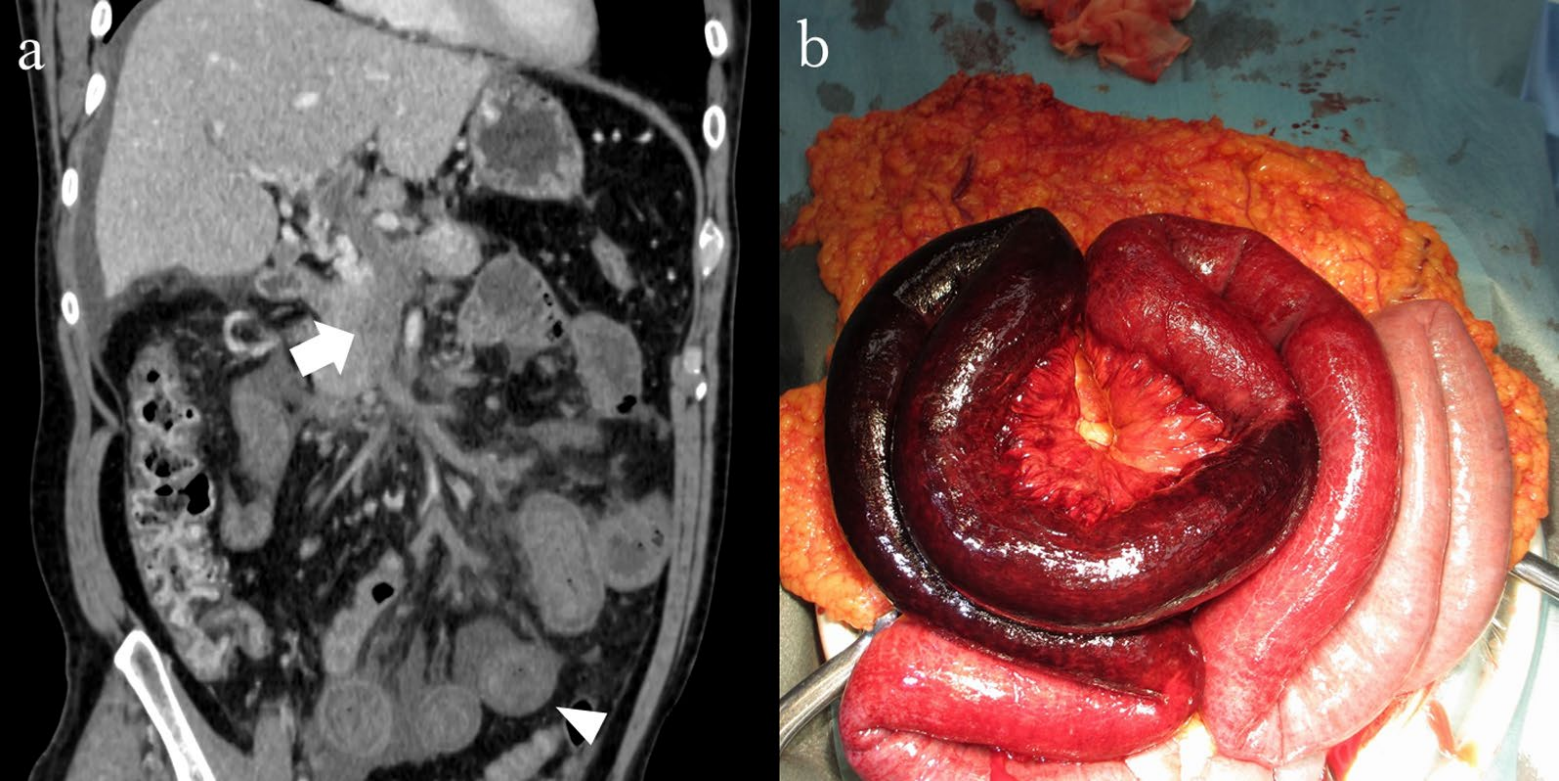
a CE-CT findings (coronal view) of Case 2. Thrombus completely occludes SMV and portal vein (arrow). Jejunal wall with poor contrast enhancement and massive ascites are observed (arrowhead). b Intraoperative findings. A gangrenous small bowel measuring 160 cm is resected
